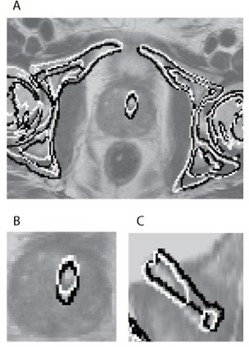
The two contours are obtained from a bone and prostate stent segmentation in CT followed by an inverse transformation of the registration by the automatic (white) and the manual (black) registration for one patient. The segmentations are superimposed on the original $T_{2}-\text{weighted}$ MR images: (a) bones and prostate stent segmentation in the axial plane; (b) region of interest and the prostate stent in the axial plane; (c) region of interest and the prostate stent in the sagittal plane. It can be noticed, in particular in image (a), that both methods are based on a registration of the prostate stent and a tightly surrounding volume as either the black or white contour follow the contour of the pelvic bone. This results in an accurate alignment of the prostate stent in image (b), which indicates that the prostate has moved relatively to the pelvic bones. In image (c), a gap in the segmentation of the prostate stent can be observed from the automatic registration caused by interpolation.
